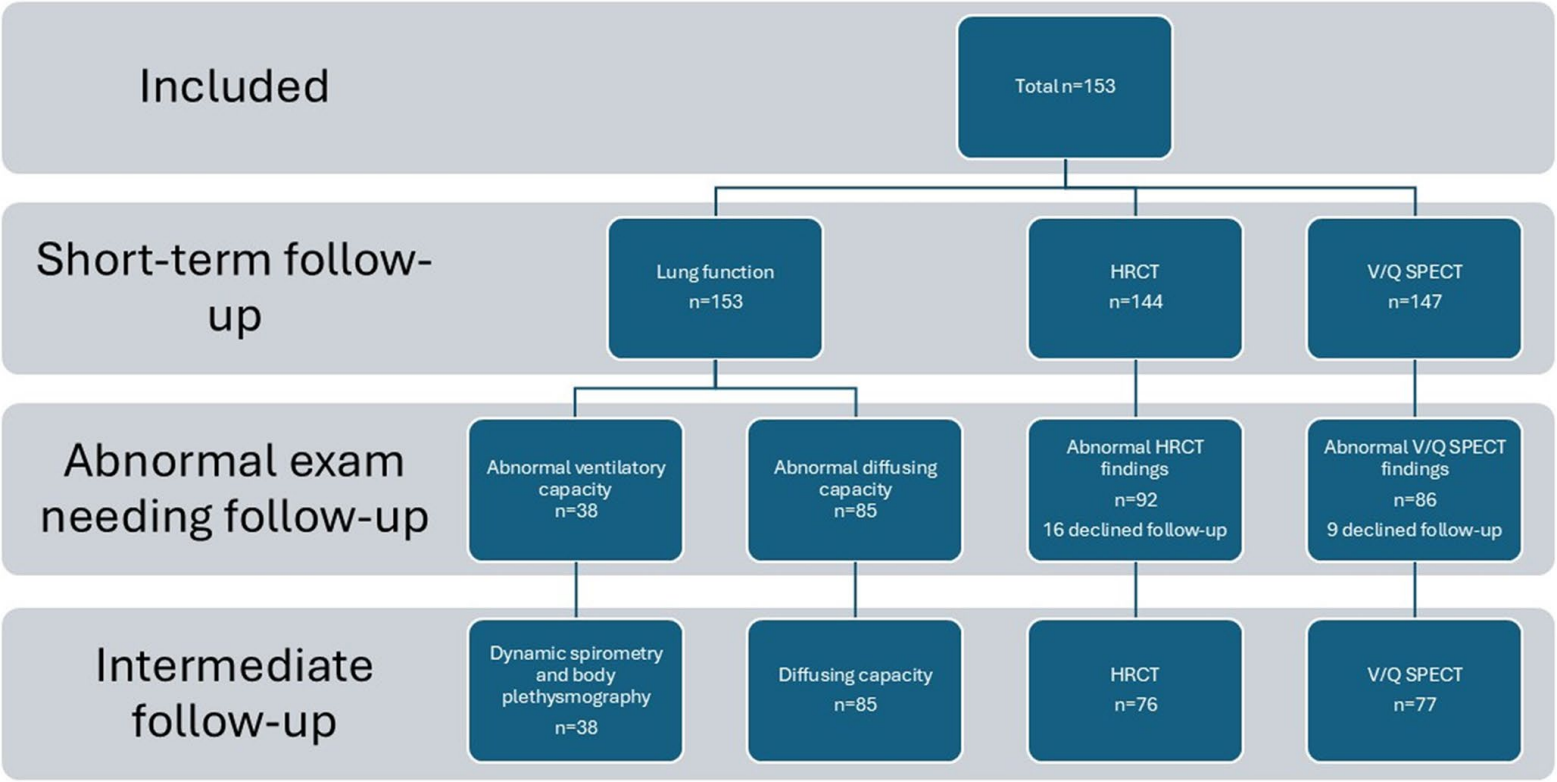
Study overview. Number of study participants from the SECURe study that underwent lung function testing, chest high-resolution computed tomography (HRCT), and ventilation-perfusion single-photon emission computed tomography (V/Q SPECT) at short-term and intermediate follow-up after COVID-19
